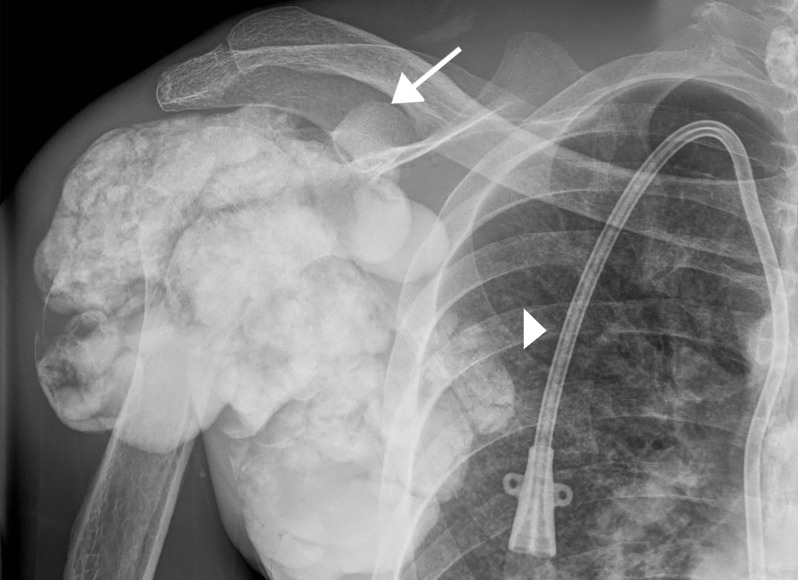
Right shoulder radiograph AP radiograph of the right shoulder demonstrating large, dense, lobulated calcifications in a periarticular distribution (arrow). Double lumen dialysis catheter (arrowhead).
